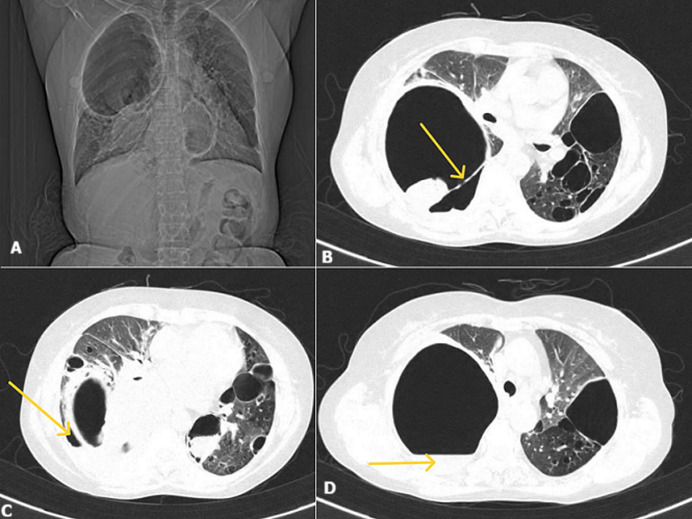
A) chest X-ray showing large right-sided bulla; B) high-resolution computed tomography thorax showing thick wall indicated with arrow; C) sign of double wall (arrow); D) water fluid level indicated with arrow
